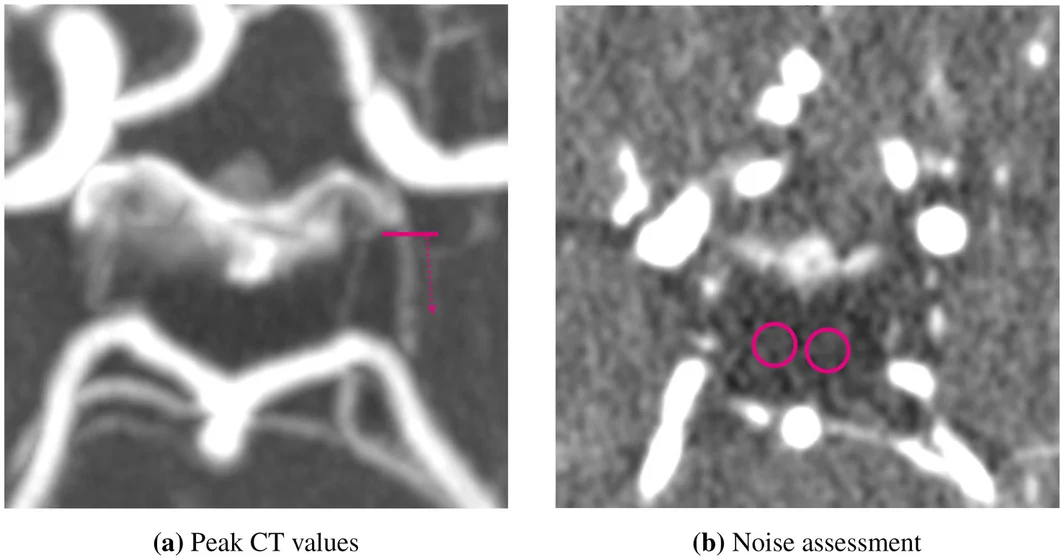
Quantitative assessment methods for CTA image analysis. (a) Measurement of peak CT values in the anterior choroidal artery (AChA) using profile curves. The red line indicates the measurement location along the vessel course, excluding the first 2 mm from the origin to avoid partial volume effects. (b) Noise evaluation method using standard deviation measurements in the basal cistern. Two circular regions of interest (ROIs) of 10 mm^2^ (red circles) were placed in homogeneous areas, and the average standard deviation was calculated.
